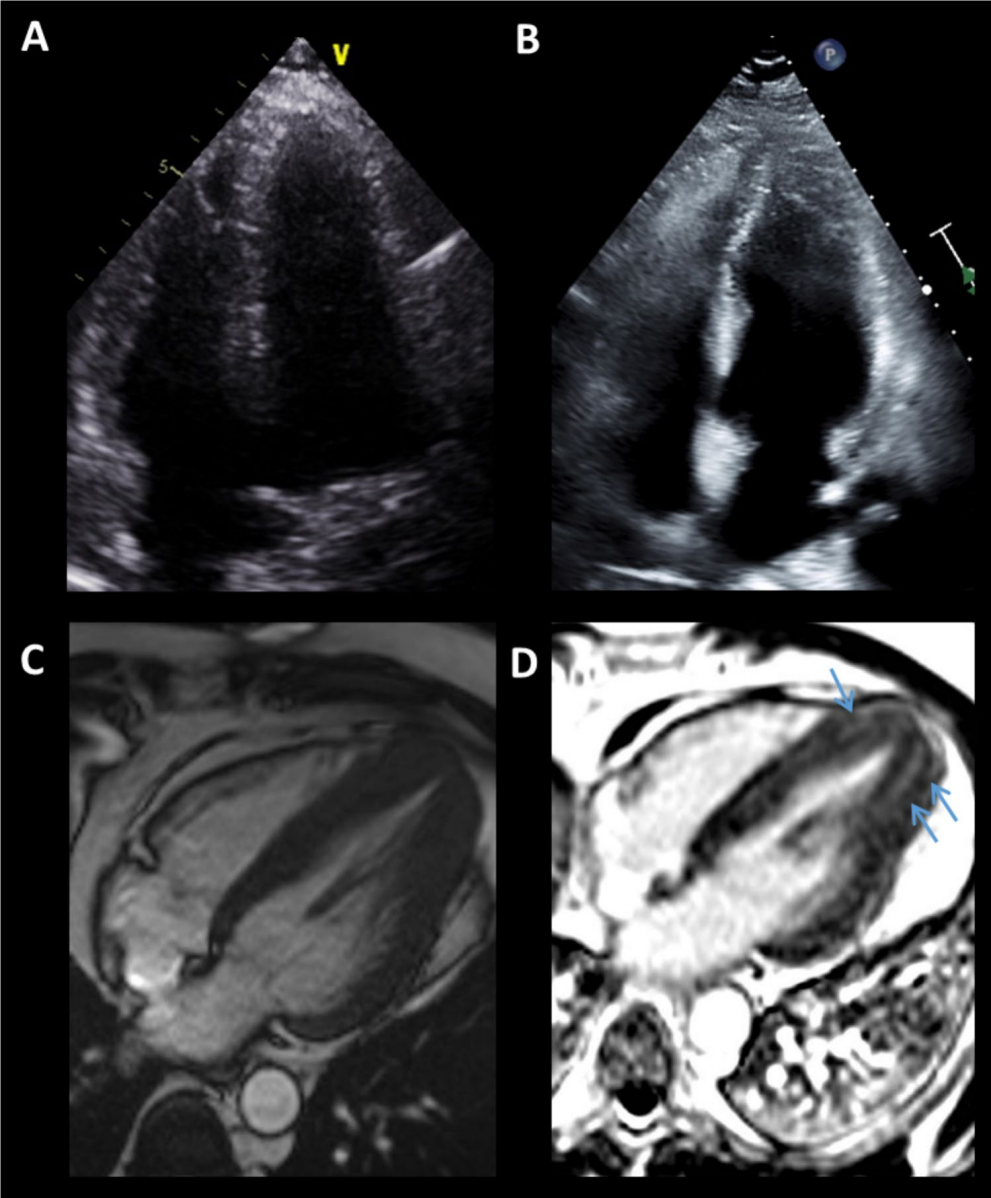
Apical Hypertrophic Cardiomyopathy. Apical hypertrophic cardiomyopathy was not seen on the initial echocardiography (A) but later diagnosed on a repeat study a year later (B). Cardiac magnetic resonance imaging confirmed the diagnosis by demonstrating the “spade-like” apex on cine (C) and patchy fibrosis in the hypertrophied segments (*blue arrows*) on late gadolinium enhancement imaging (D)
